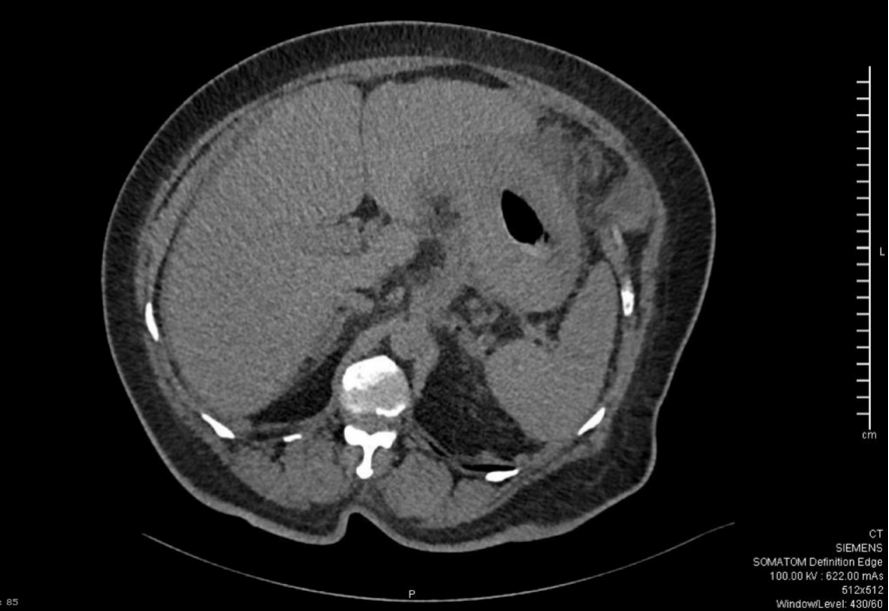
Computed tomography scan showing diffuse thickening of the stomach wall
